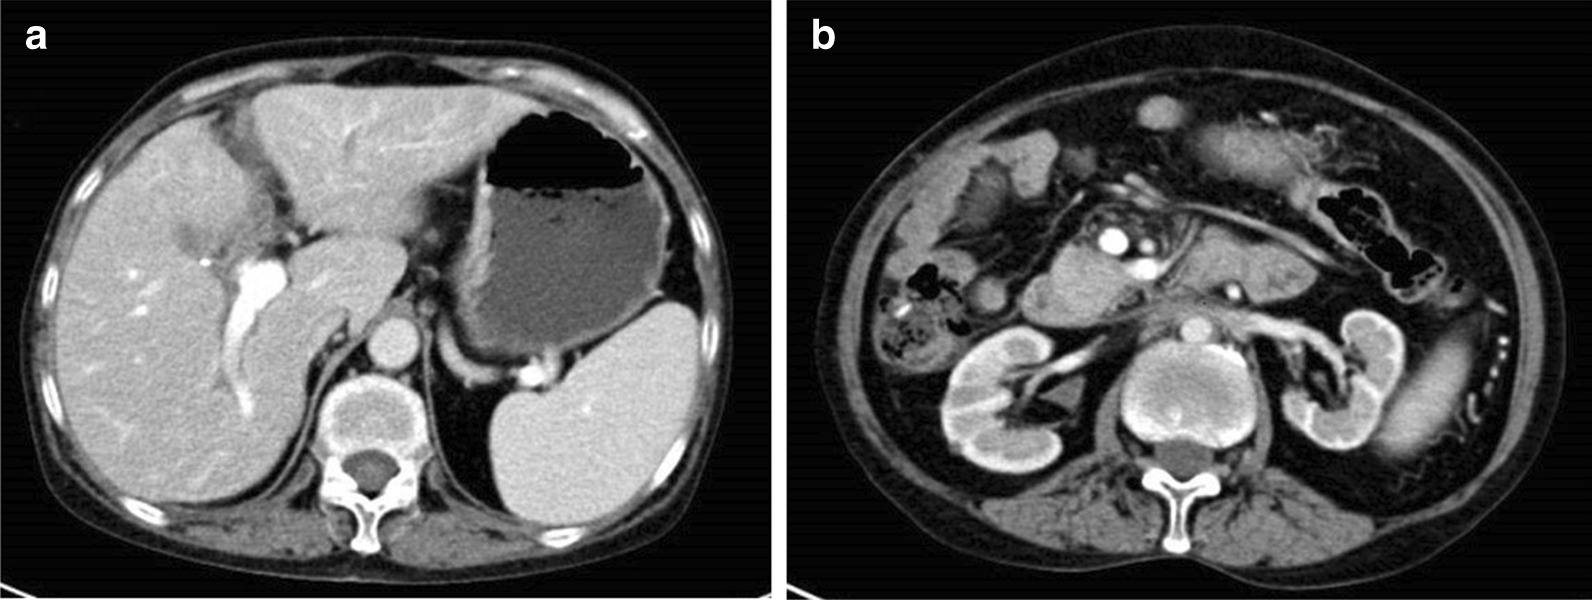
Repeat abdominal CT. a Dilatation of the intrahepatic and extrahepatic bile ducts. b Effusion of the bilateral upper ureter
